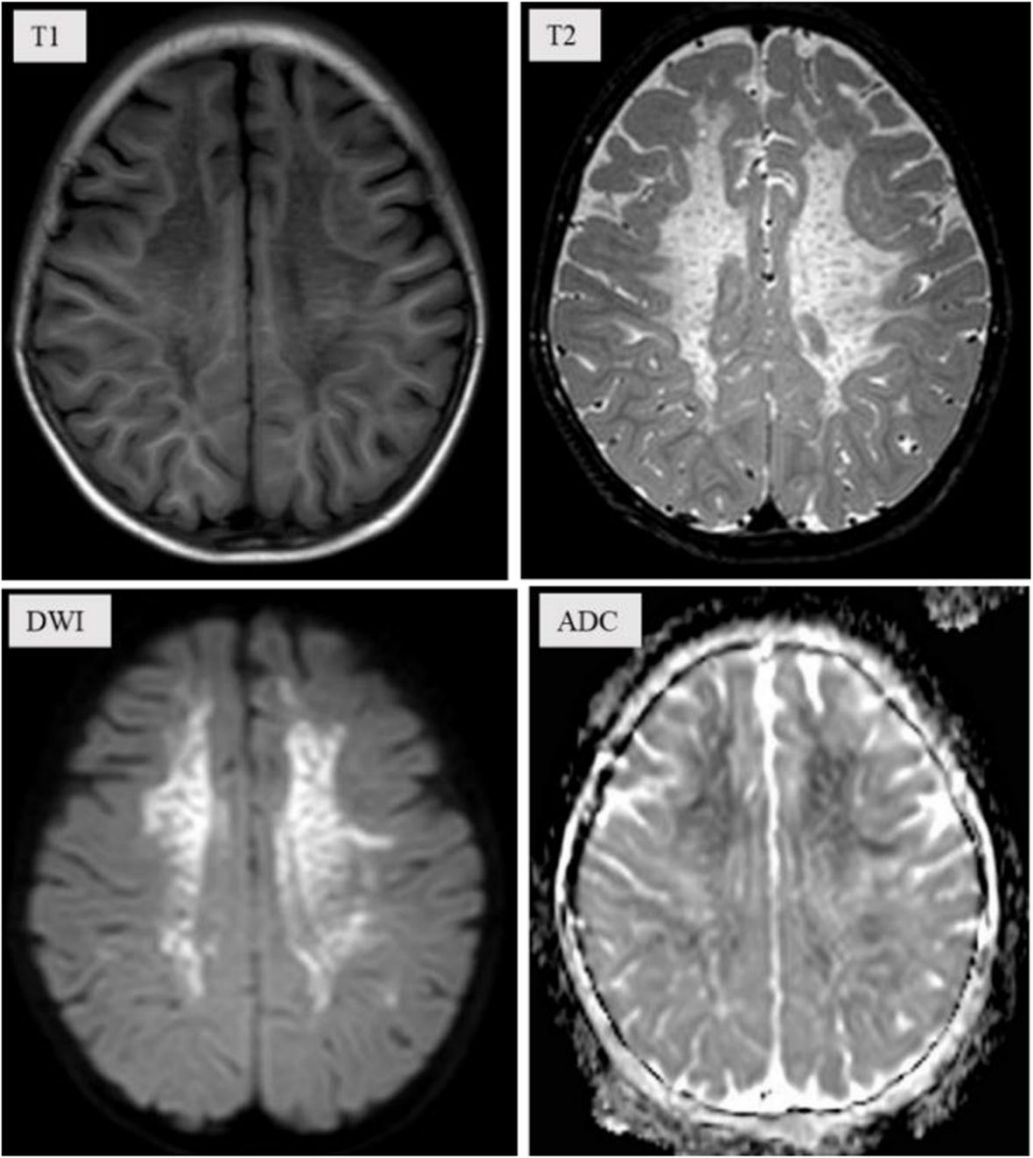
Brain MRI of showed "leopard skin sign" within the cerebral white matter
